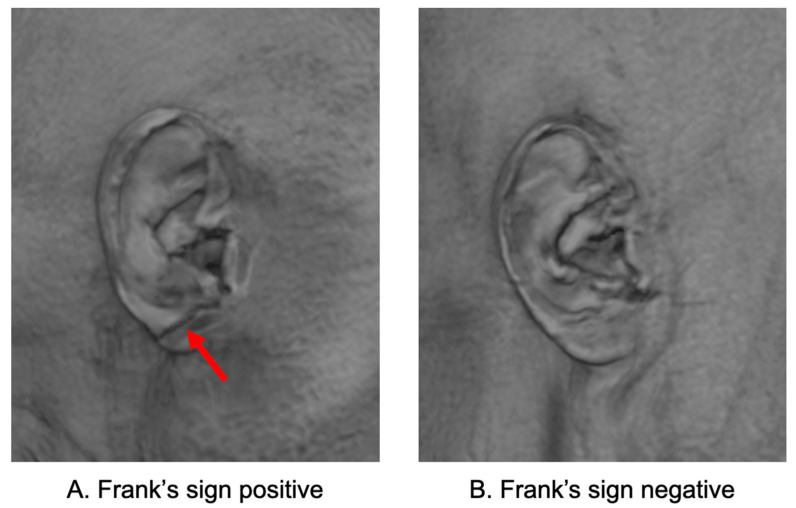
MRI-based visualization of Frank’s sign. (A) FS-positive: A diagonal earlobe crease extending from the tragus to the outer ear border (arrow). (B) FS-negative: Earlobe without a diagonal crease. Images were derived from 3D surface reconstruction of T1-weighted MRI. FS, Frank’s sign.
